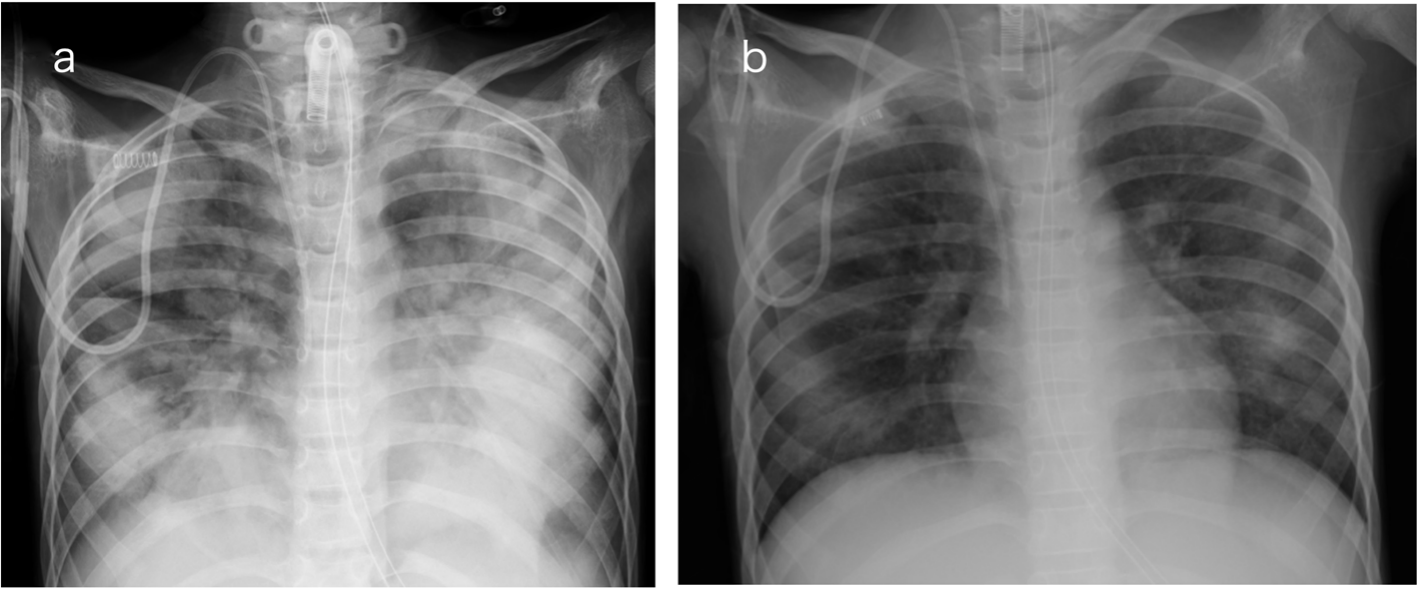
Chest radiography images of Case 2. a After tracheostomy with pulmonary edema. b Forty-eight hours after onset and treatment of pulmonary edema
